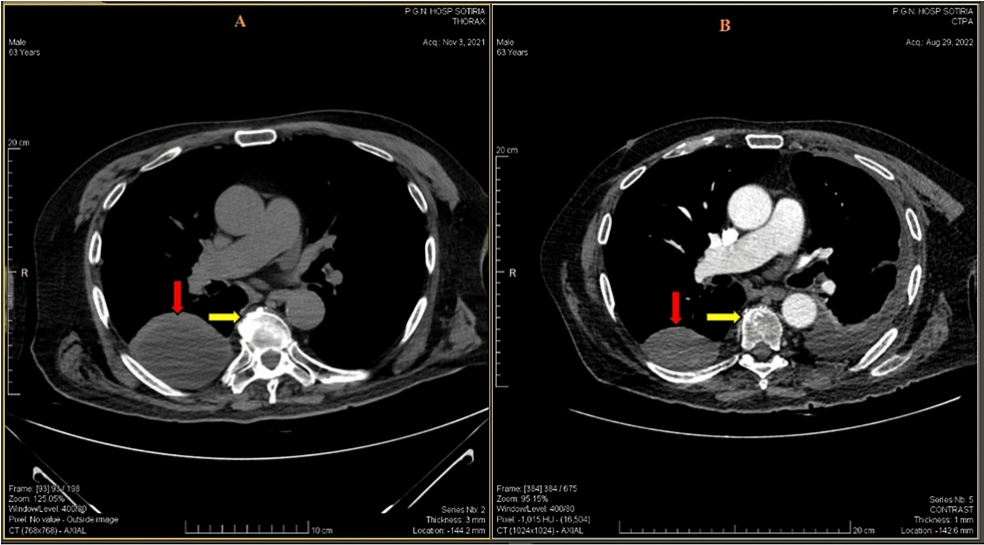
Thorax CT scans. A) Pre-treatment imaging shows a Th12 spinal lesion (yellow arrows) and paraspinal fluid collection (red arrows) and B) imaging nine months after treatment indicates a decrease in the spinal lesion (yellow arrows) and paraspinal fluid collection (red arrows) CT: Computed tomography
